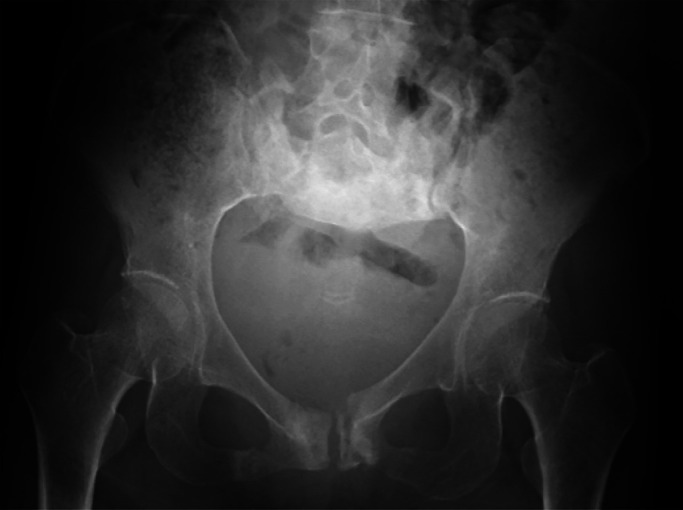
X-ray of pelvis on admission to our hospital; 3 months after the first injury.
